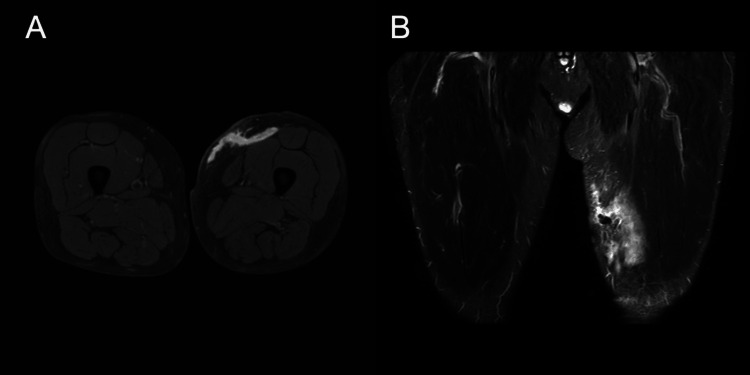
MRI on day 14 after discharge Axial T1 fat suppression MRI shows a hyperintense hematoma between the subdermal fat and fascia (A). Coronal short tau inversion recovery MRI shows a longitudinal, hyperintense hematoma about 100 mm in length between the subdermal fat and fascia (B). Hematoma was smaller than before because exudate and clots were extracted from two holes that had been necrotic plaques two days earlier in both A and B. MRI: magnetic resonance imaging
